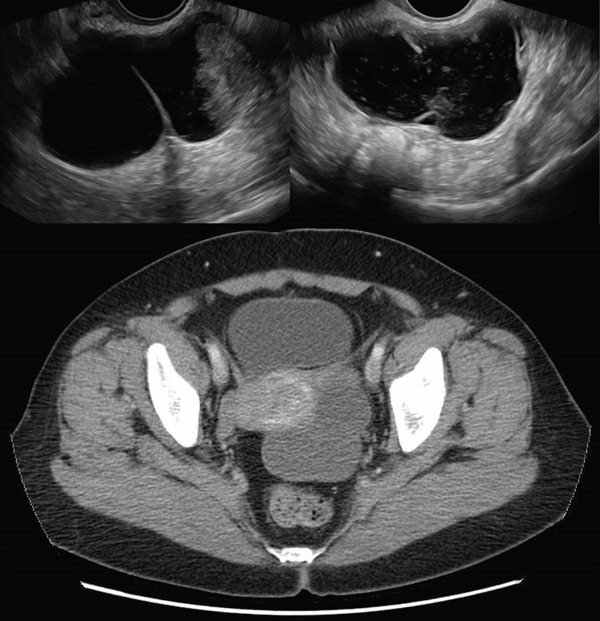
Case 2: Ultrasound and CT abdomen and pelvis of left adnexal mass.
